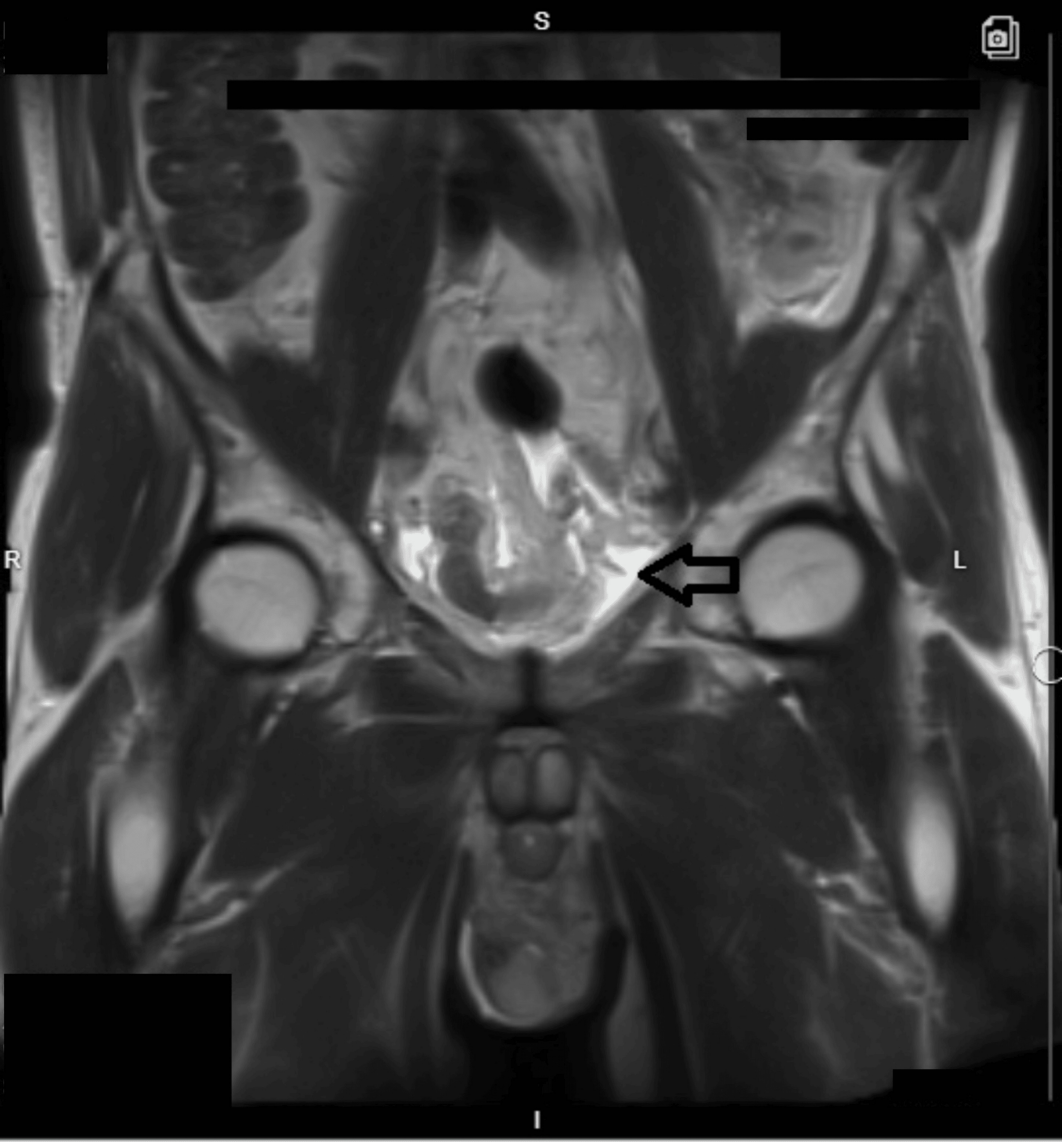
MRI of the abdomen and pelvis (T2-weighted coronal view) MRI: magnetic resonance imaging
